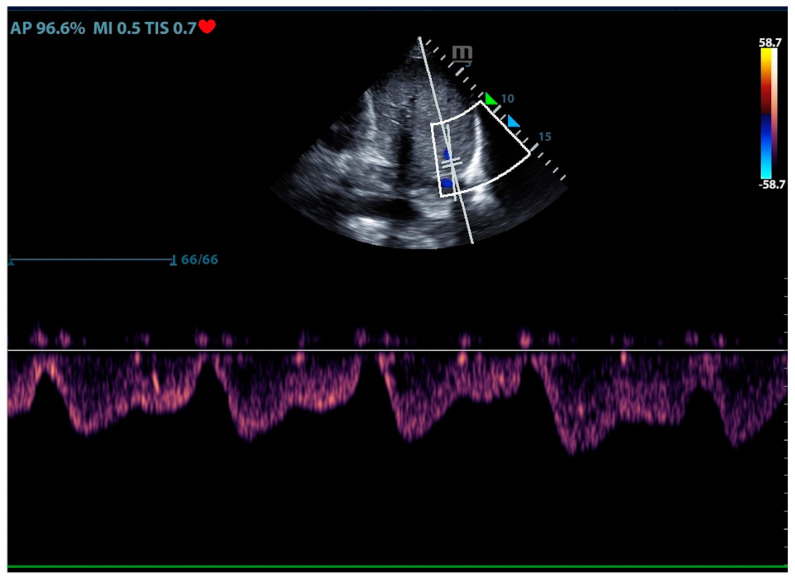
The ultrasound sign of hepatic venous excess waveform.
